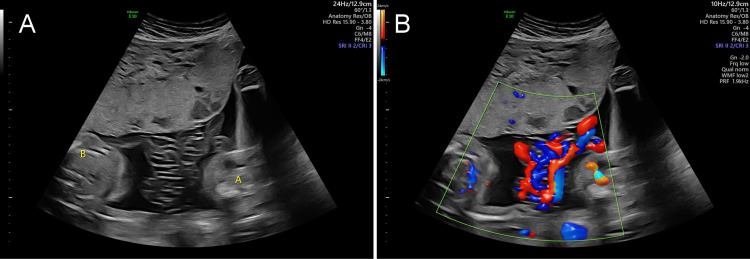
Early second-trimester ultrasound demonstrating significant cord entanglement, without color doppler in (A) and with color doppler in (B)
